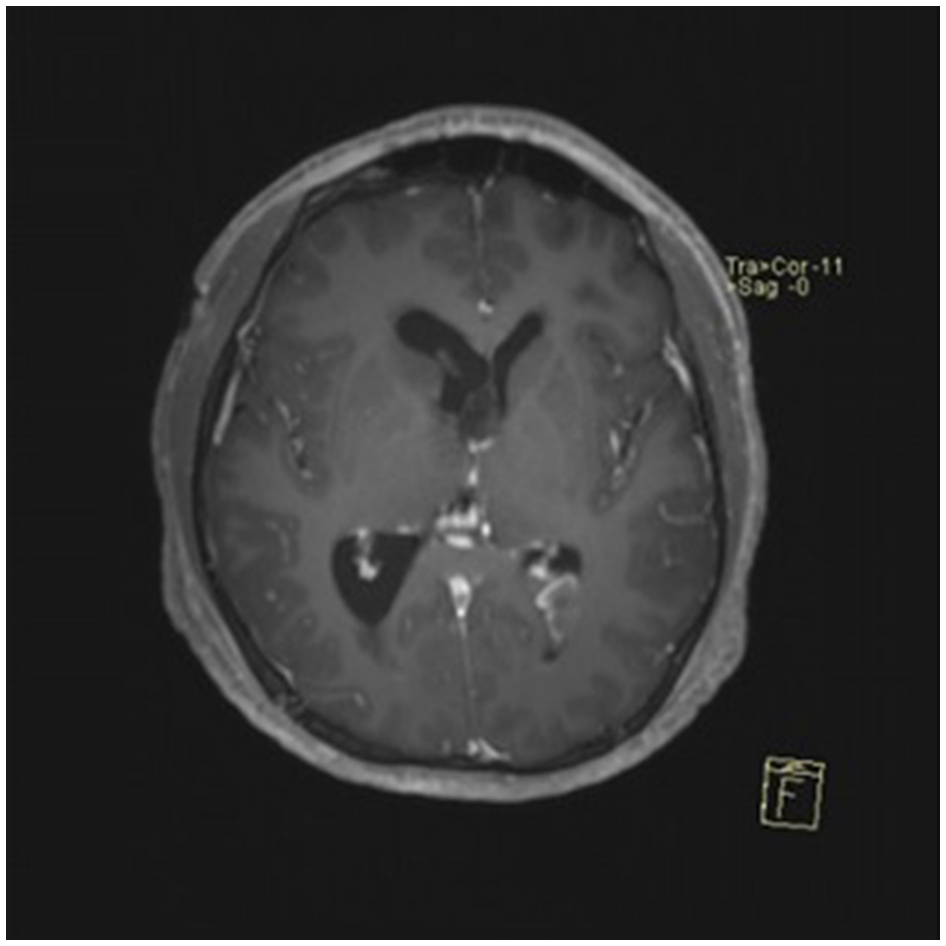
Postoperative day 1 contrast-enhanced MRI (axial view) showing complete tumor removal and resolution of hydrocephalus.
